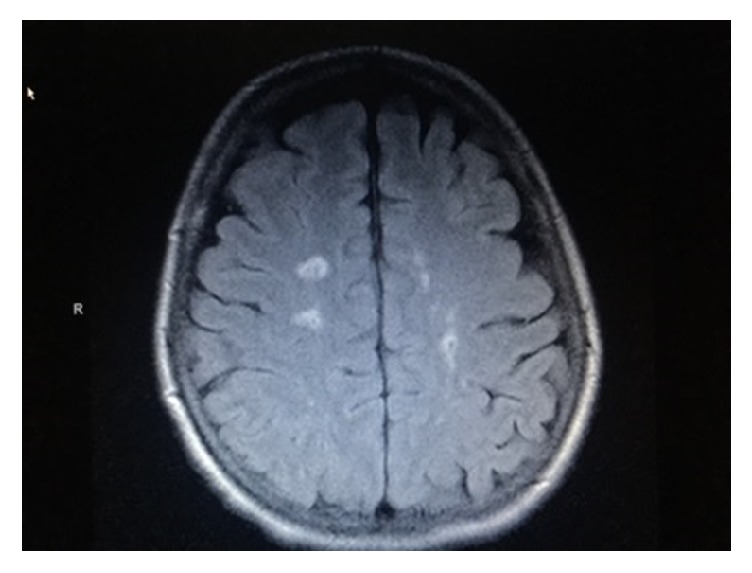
Six-week follow-up MRI brain (FLAIR) showing improvement in bilateral hyperintense lesions.
